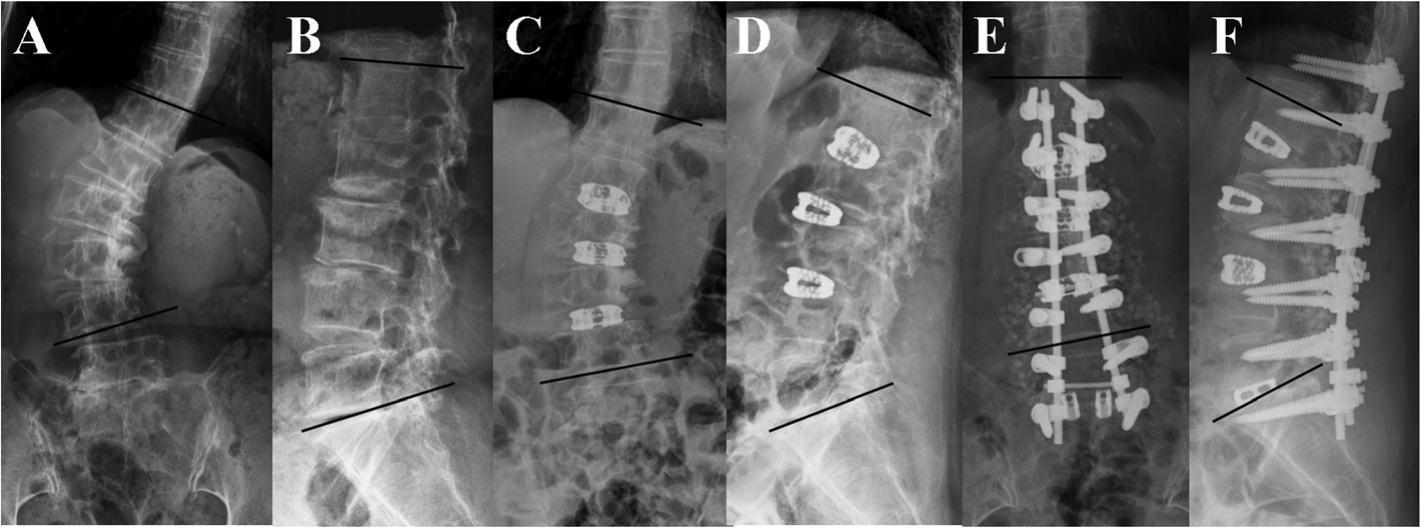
A 64-year-old woman complained low back pain with bilateral sciatica and claudication for several years. Radiographs of anteroposterior view (A) and lateral view (B) showing degenerative lumbar scoliosis from T12 to L5 with lateral bridged traction vertebral osteophytes over L2-3,L3-4 associated with severe disc space narrowing over L1-2 ,L2-3. After anterior lumbar interbody fusion with three SynCages over L1-2, L2-3, and L3-4, the scoliotic angle (T12-L4) was improved from 37° to 17° (C) and the lumbar lordotic curve was improved from 4° to 29° (D). One week later, posterior instrumentation of T12-S1 with posterior interbody fusion of L5-S1 was performed. The scoliotic angle was improved from 17° to 6° (E) and the lumbar lordotic curve was improved from 29° to 36° (F).
